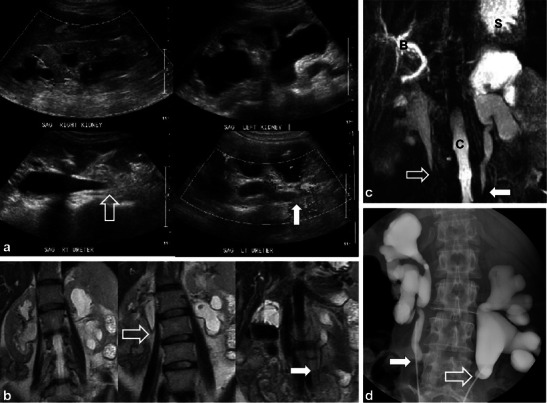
A 52-year-old woman with metastatic appendiceal mucinous adenocarcinoma treated with prior surgery and chemo-RT. Sagittal ultrasound images (a) show bilateral hydroureteronephrosis with smooth, tapered, transition of the right (*open white arrow*) and the left (*solid white arrow*) proximal ureters. Coronal T2 ssFSE (b) images confirm the presence of bilateral hydroureteronephrosis and smooth, tapered narrowing of both proximal ureters (*right and left*—*open and solid white arrows*). Coronal maximum intensity projection (MIP) image from heavily weighted T2 coronal 3D MRCP (c) also depicts the bilateral hydroureteronephrosis and the areas of transition in the proximal ureters (*open and solid white arrows*) (*B* common bile duct, *S* stomach, *C* spinal canal). Overhead images (d) from bilateral retrograde urethrogram (RUG) demonstrates correlation of findings between modalities with classic features of radiation induced ureteric strictures again noting smooth, tapered margins (*open and solid white arrows*) of both ureters
